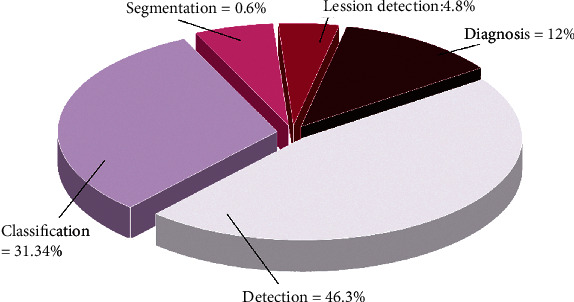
X-ray image analysis objectives.
